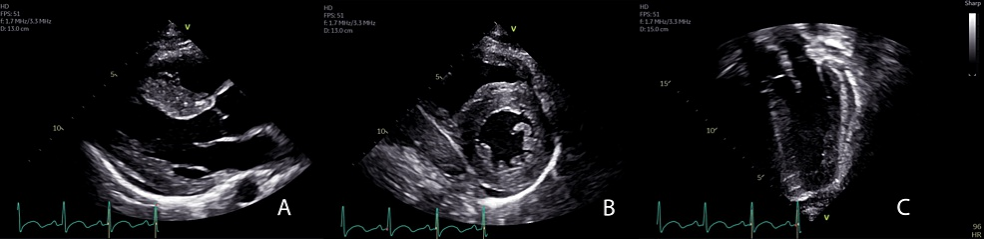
Echocardiogram demonstrating global left ventricular hypertrophy Transthoracic echocardiography of the parasternal long axis (A), parasternal short axis (B), and apical three-chamber view (C) were obtained on day eight of hospitalization while undergoing treatment for MIS-C. There was global hypertrophy of the left ventricle.
